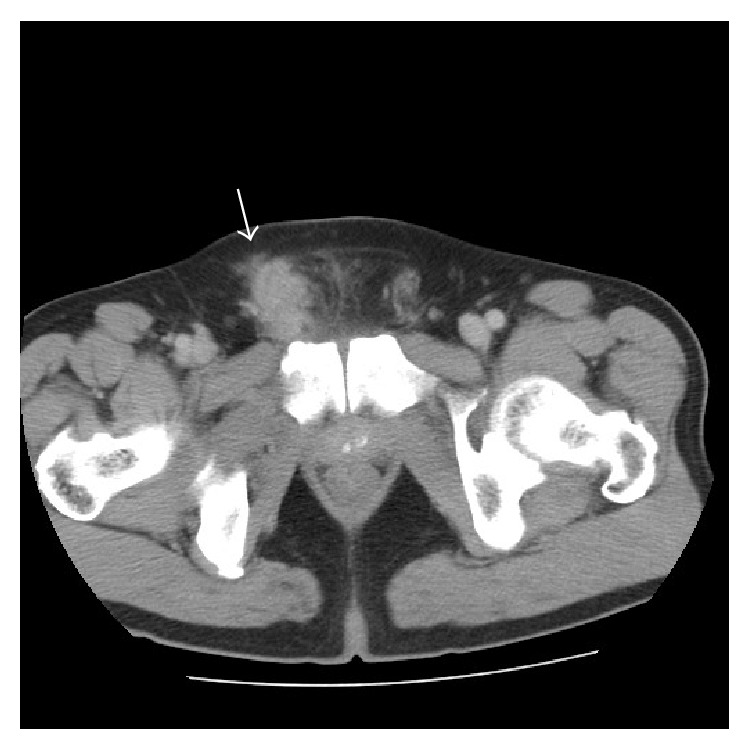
Abdominal CT. Abdominal CT reveals a 3.7 cm diameter slightly enhanced tumor (arrrow) in the right spermatic cord.
